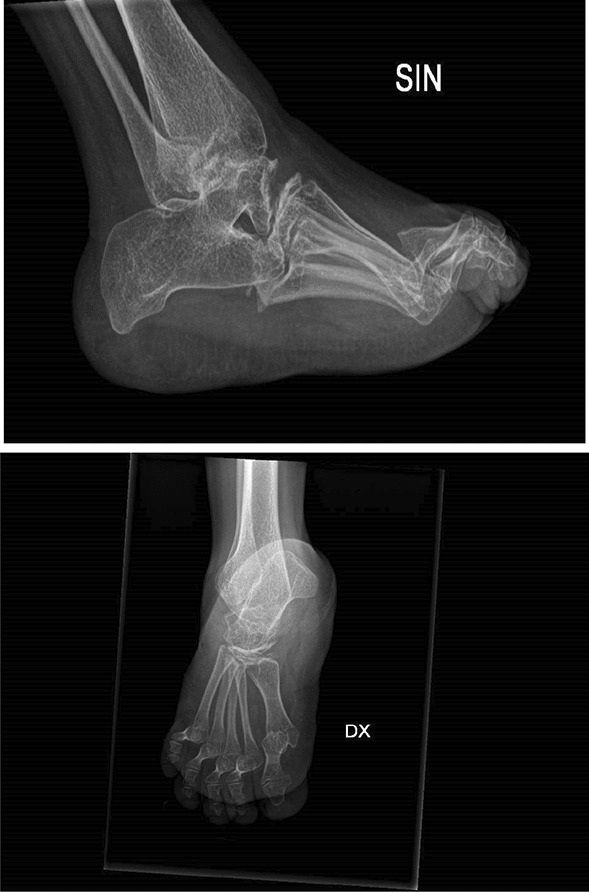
Pt. 2 X-ray at age 16y 6m. Feet radiograph showing complete resorption of tarsal bones and generalized osteopenia
